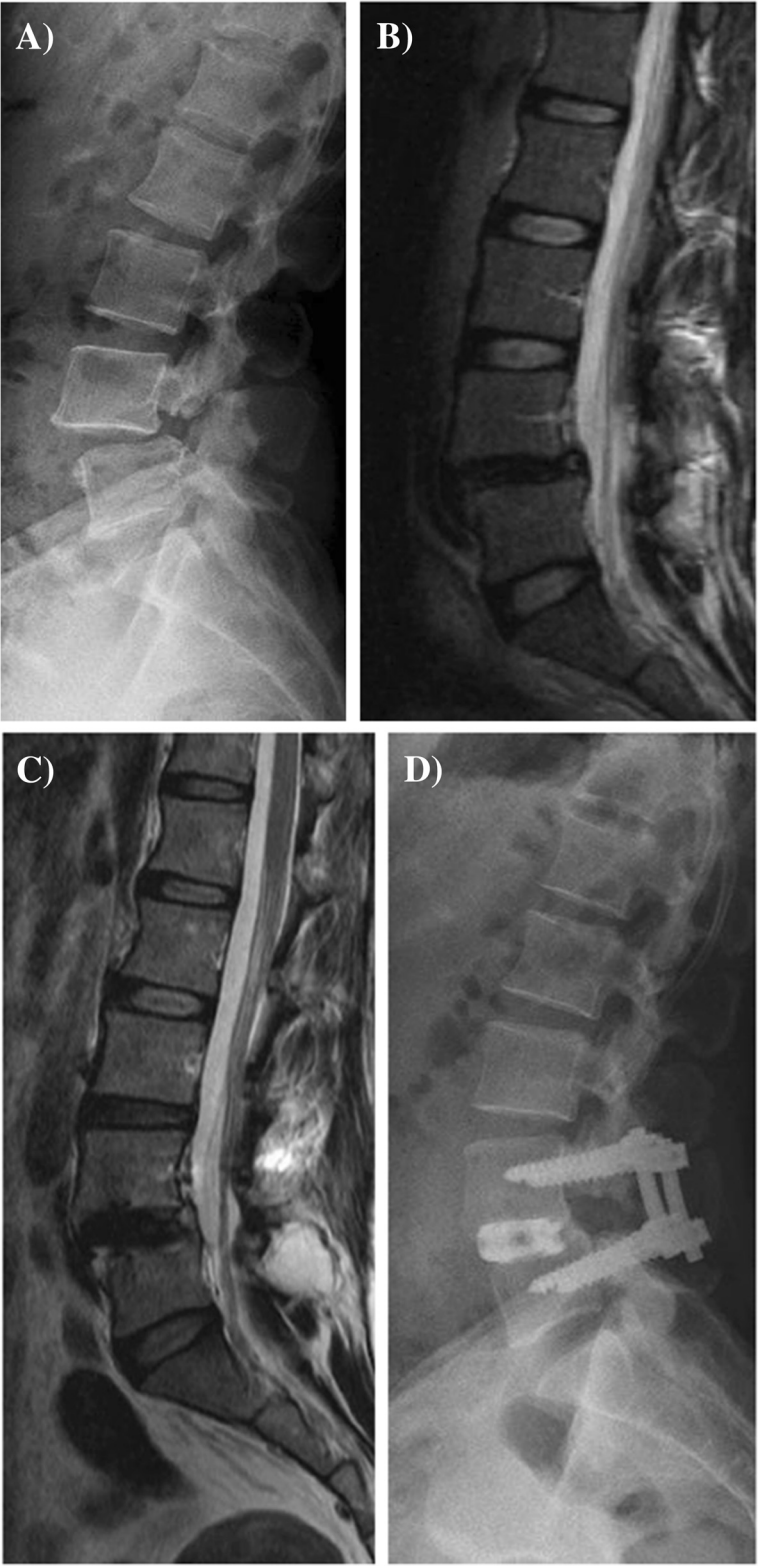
Imaging of isthmic spondylolisthesis in a 49-year-old woman. (A) Lateral radiography reveals L4-5 isthmic spondylolisthesis. (B) T2-weighted sagittal magnetic resonance imaging (MRI) reveals L4-5 spondylolisthesis without degeneration at the L5-S1 level. (C) Sagittal MRI reveals reduced slippage at the L4-5 level and no acceleration of degeneration at the L5-S1 level after minimally invasive transforaminal lumbar interbody fusion at L4-5 (5 years after surgery). (D) Seven years later, lateral radiography reveals complete interbody fusion of L4-5 and good maintenance of disc height at the L5-S1 segment.
